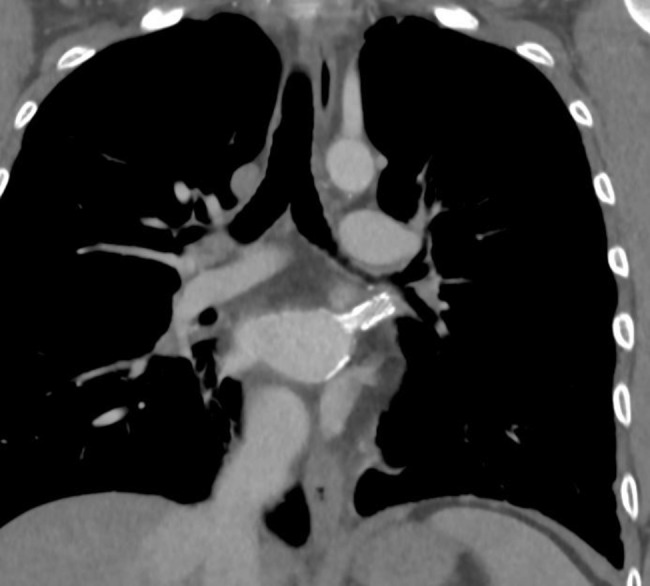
CT showing the common ostium of the left PVs after stenting
